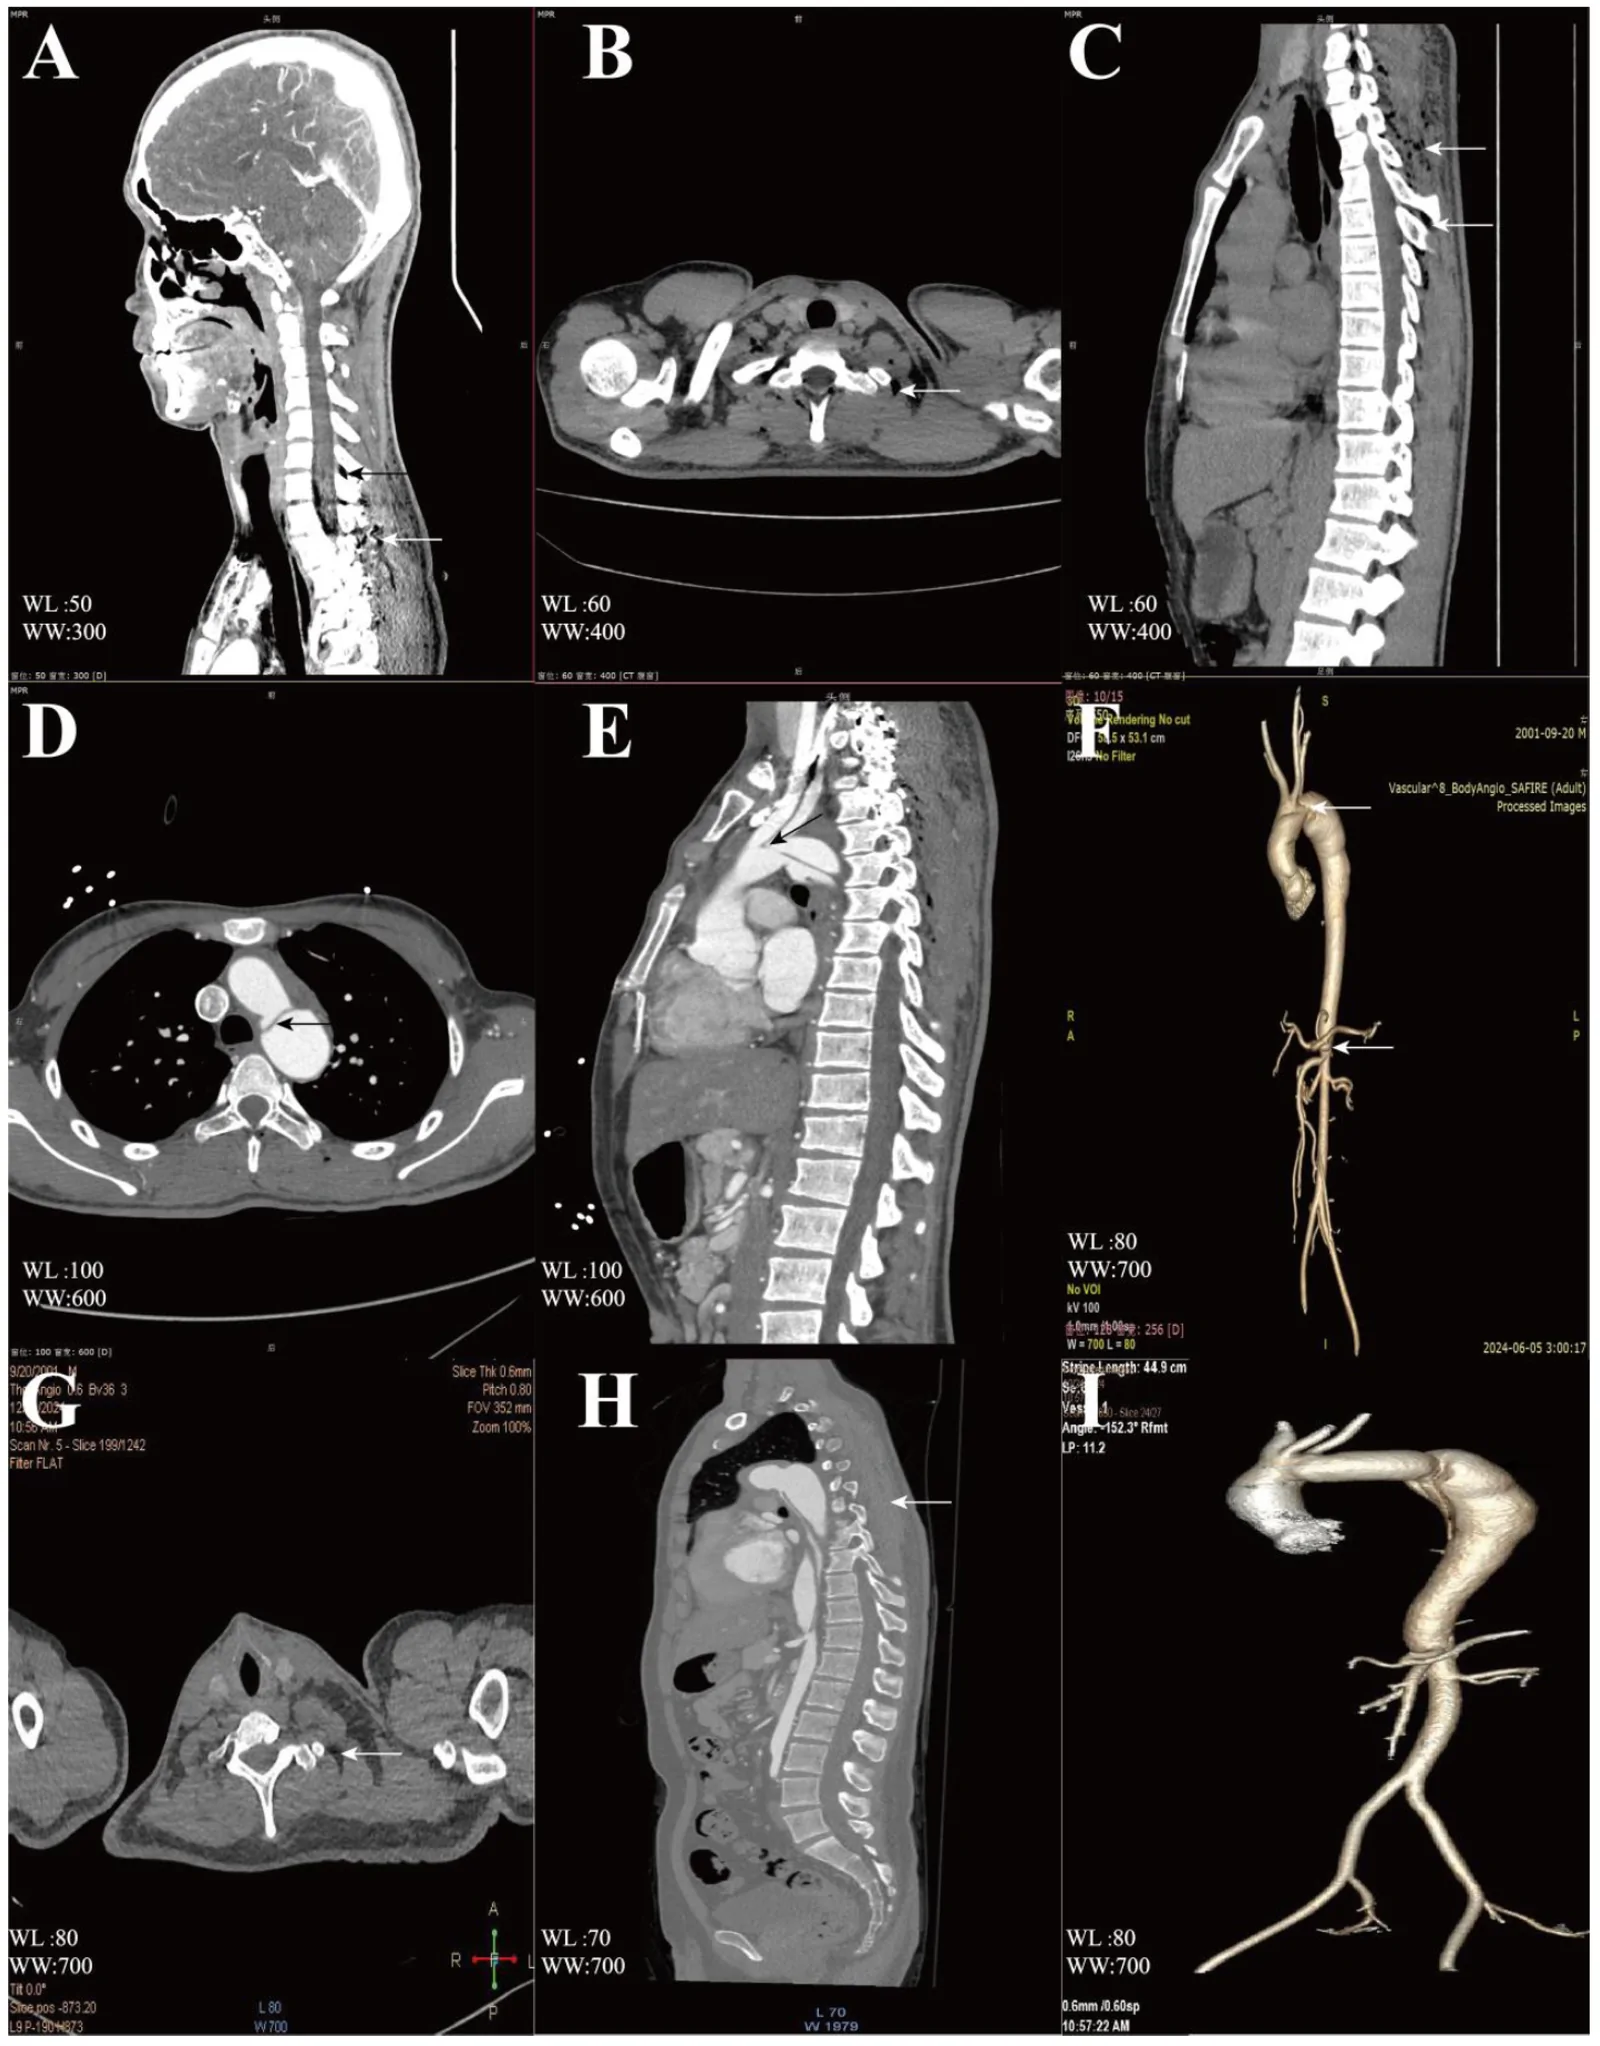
In CT scans, WL means window level and WW means window width. The presence of emphysema in the neck(between the two spinous processes (A), beside of transverse process (B), and intermuscular and subcutaneous air in the back (C); CT angiography of the thoracic and abdominal aorta: The descending aorta showed a double lumen image with a smaller true lumen and larger false lumen (D). The intimal rupture is located in the aortic arch, between the left common carotid artery and the left subclavian artery, and the width of the rupture is about 7mm (E). The dissection extends down to the upper part of the abdominal aorta, above the opening of the superior mesenteric artery; The left common carotid artery is of common origin with the brachiocephalic artery (F).The emphysema disappeared in the neck (G) and back (H). The 3D reconstruction showed that the artificial blood vessel healed well (I).
